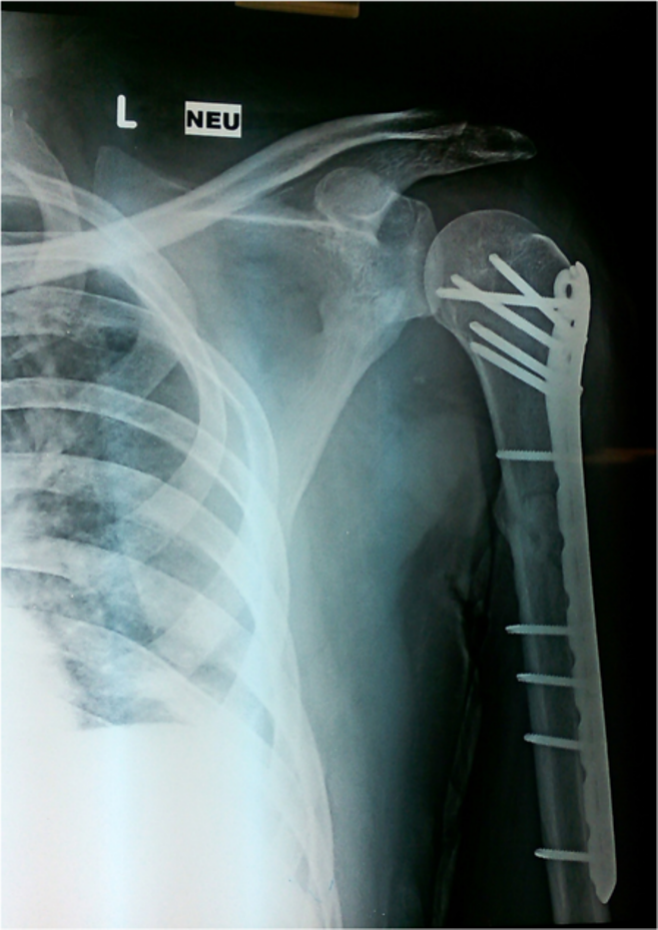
Radiograph showing callus formation at the fracture site.
